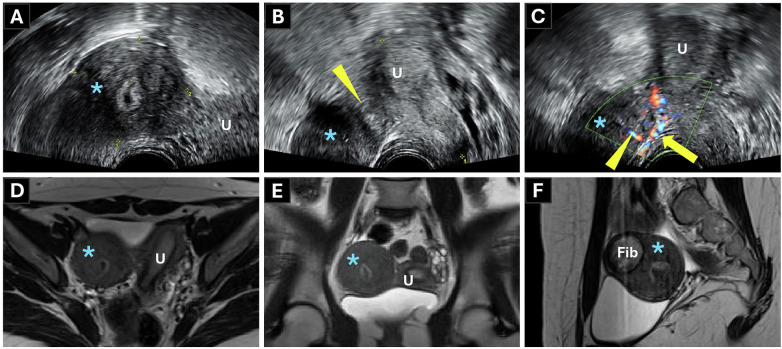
Preoperative multimodal imaging. (A–C) transrectal ultrasound (TRUS) findings. (A) Transverse view delineating the left-sided hemiuterus (U) and the adjacent rudimentary horn with hematometra (star). (B) The fibromuscular band (arrowhead) connecting the two structures. (C) Color Doppler imaging demonstrating a separate arterial branch (arrowhead) from the main uterine vessels (arrow) supplying the horn. (D–F) T2-weighted magnetic resonance imaging (MRI) scans confirming the TRUS findings. (D) Transverse view showing the hemiuterus (U) and rudimentary horn (star). (E) Coronal view confirming the absence of communication. (F) Sagittal view demonstrating the associated fibroid (Fib).
